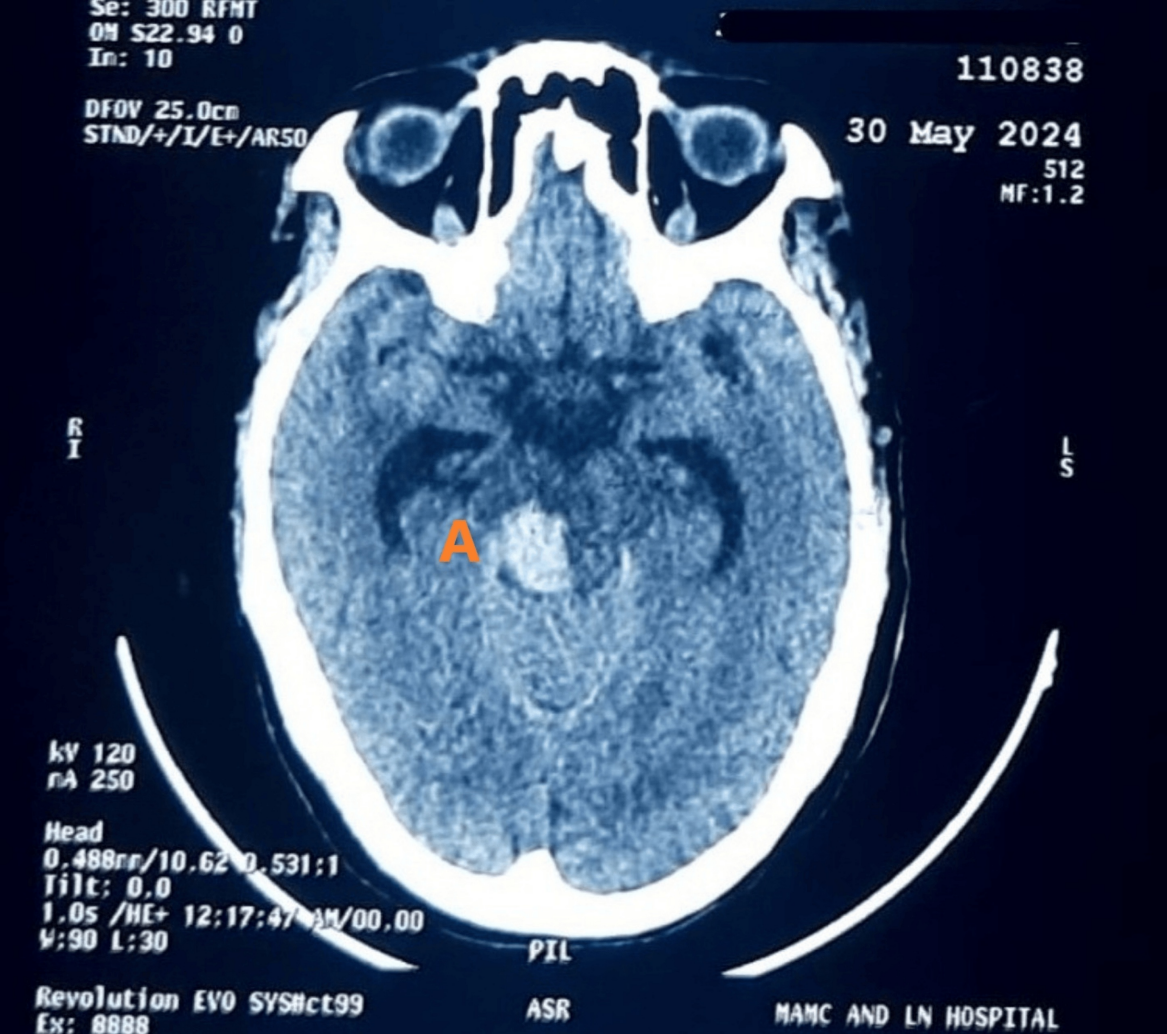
NCCT of the head following altered sensorium showing pontine hemorrhage on the right side (labelled as A) NCCT: non-contrast computed tomography
